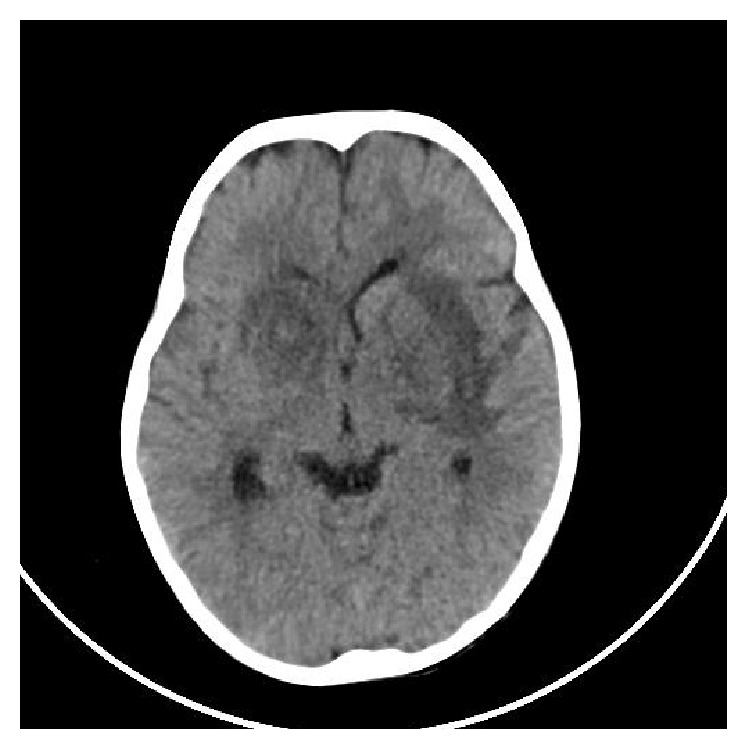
Axial brain CT image (noncontrast) of patient 2 showing hypodense areas in both thalami and basal ganglia.
